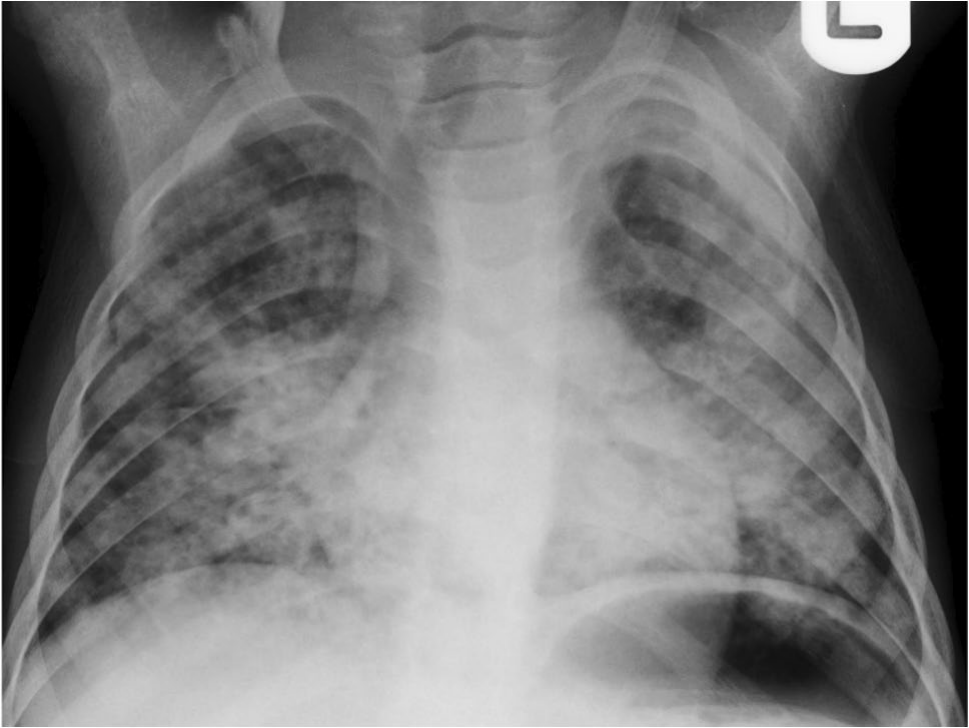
Posteroanterior chest radiograph of an 8-month-old girl with proven tuberculosis (TB) shows multiple ill-defined nodules of differing sizes and parenchymal opacification of the right middle and both lower lobes. These features are suggestive of bronchogenic spread of TB
